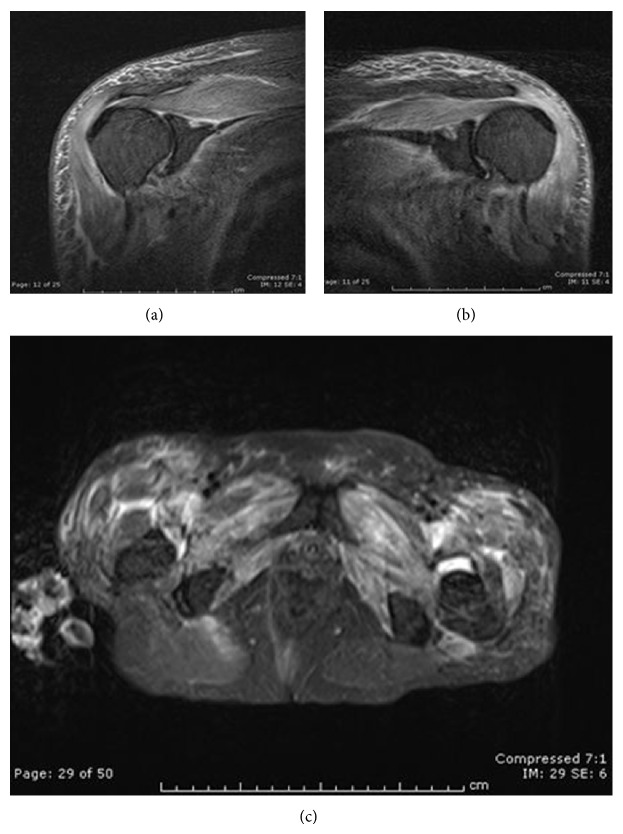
MRI with short tau inversion recovery (STIR) image showing diffuse symmetrical proximal muscle edema. (a) Right shoulder, (b) left shoulder, and (c) pelvis.
